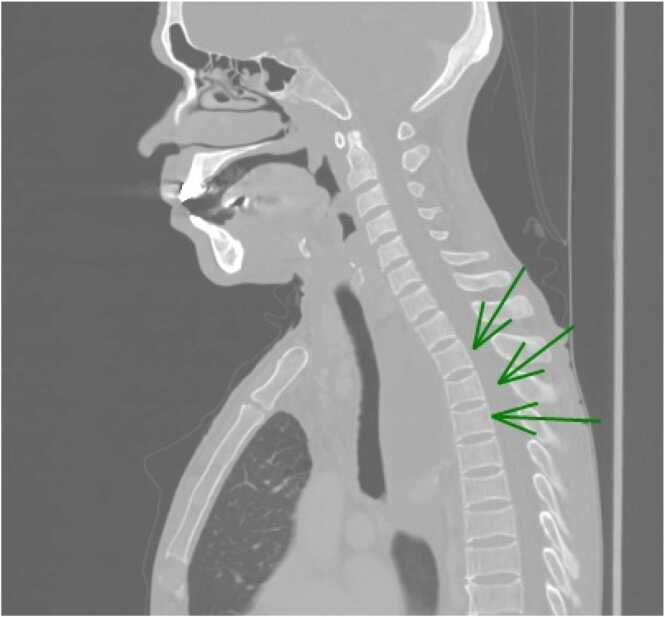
Sagittal section of a contrast-enhanced CT scan after dilation of the stenosed vein showing the complete resolution of the bone lesions previously observed.
